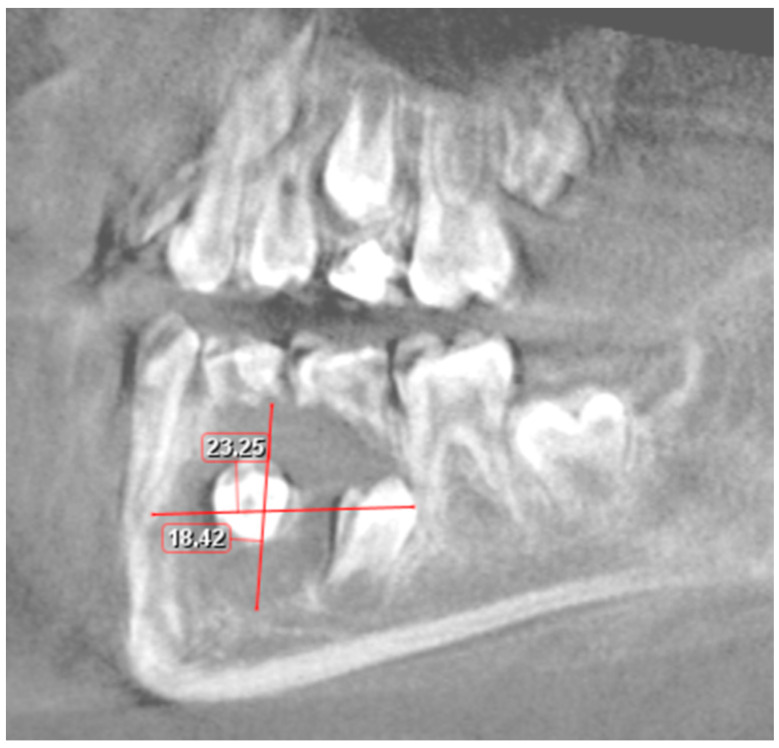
Sagittal CBCT image. Visible cyst measurements.
